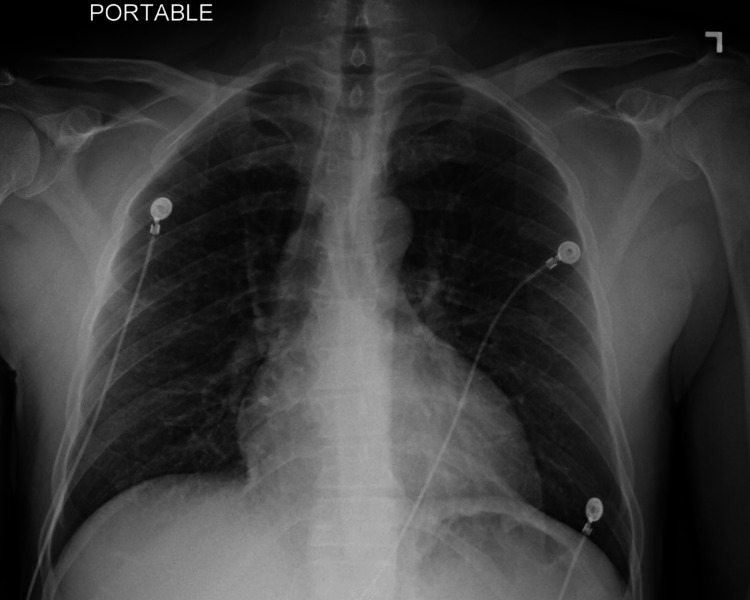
Patient’s chest X-ray one year prior to presentation The patient’s previous chest X-ray showed no anomalies of acute significance. Please note the absence of pleural effusions in this chest X-ray.
